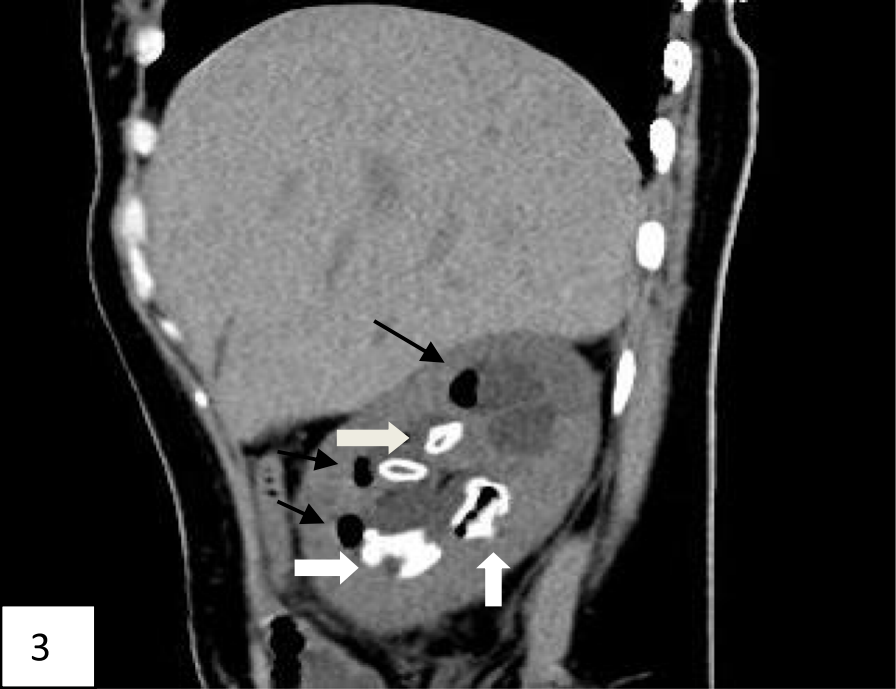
Show the non-contrast urinary CT scans during hospital admission
